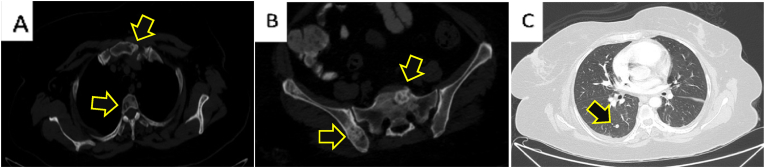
(A): is a CT scan showing lytic lesions of the spine and sternum, (B): is a CT scan showing sclerotic and lytic lesions of the pelvis, and (C): is a CT scan showing metastatic lesion in the right lung, (Arrows define the significant findings).
